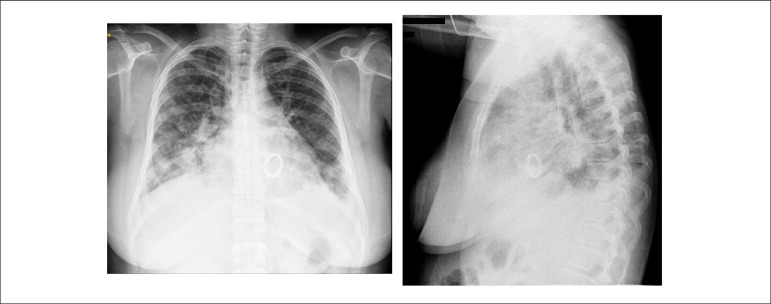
Admission chest x-ray: signs of congestion and pulmonary infection (air
